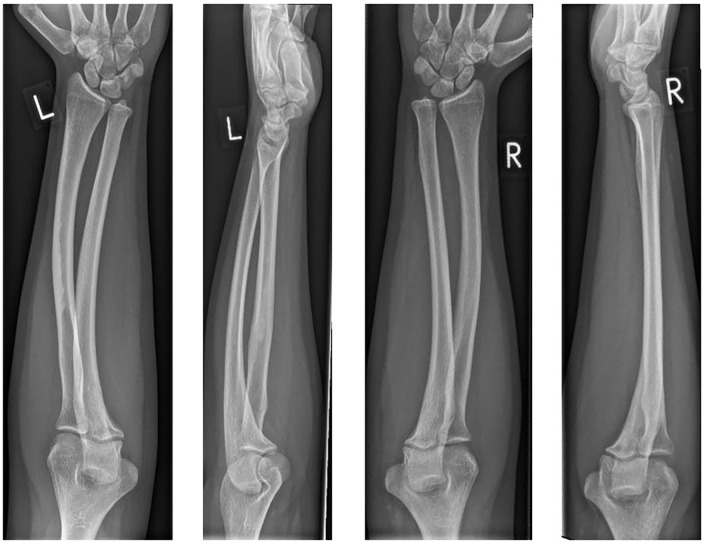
Posteroanterior and lateral radiographs of the left and right forearms (left to right) before the surgery.
